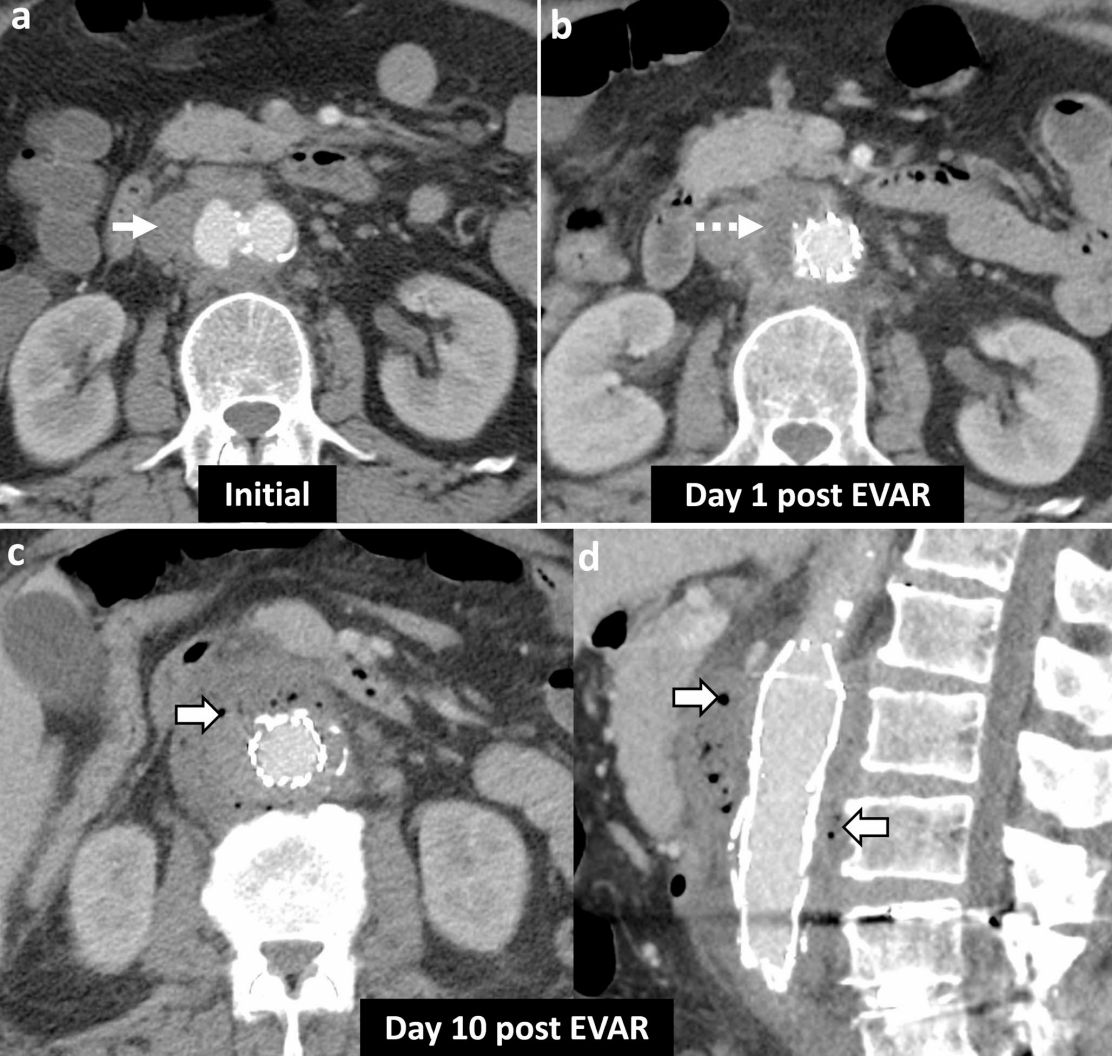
Emphysematous aortitis. A 73-year-old with pancreatic cancer and abdominal pain. CT at initial presentation a shows mycotic infrarenal aortic aneurysm with periaortic soft tissue and inflammation (solid white arrow, a). Day 1 post-EVAR CT b shows the expected aortic stent graft with peri-aortic soft tissue (dotted white arrow, b). Note the distinct absence of air on post-operative day 1. Day 10 post-EVAR CT c, d shows increased peri-aortic soft tissue with new air foci (block white arrows, c, d) suggestive of emphysematous infection. The graft was explanted, and microbiology showed *Salmonella enteritidis* graft infection
